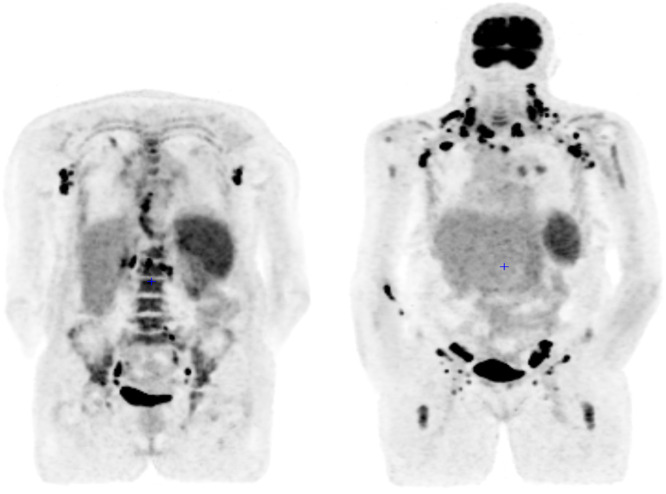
Positron emission computed tomography with ^18^F-fluorodeoxyglucose.
